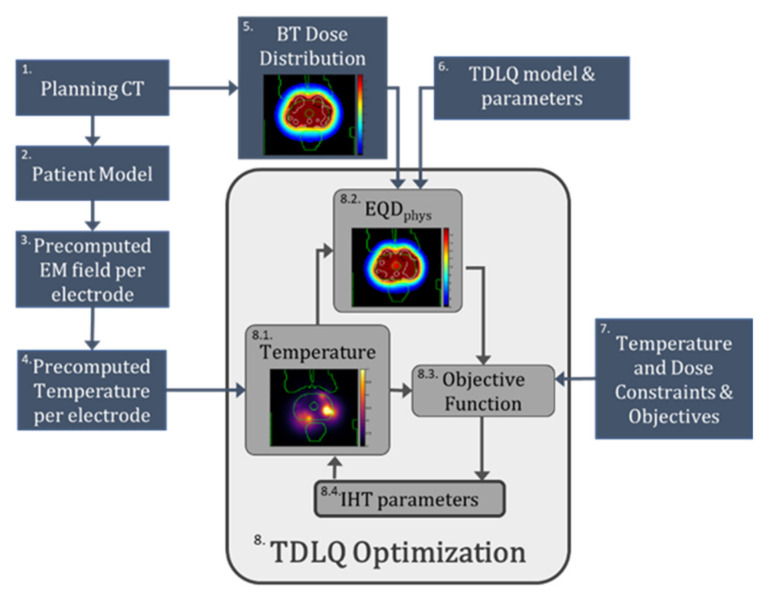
Graphical summary of the proposed optimization framework. 1. The planning CT is the initial input of the process. 2. The patient model is generated. 3. The EM field per electrode is precalculated. 4. The temperature distribution per electrode is precalculated. 5. The BT dose distribution is imported from the BT treatment planning software. 6. The TDLQ model is used for the calculation of the combined effect. 7. Both temperature and dose constraints and objectives are used for the optimization process. 8. The TDLQ optimization process optimizes the IHT parameters (8.4) that generate a temperature distribution (8.1) from which an EQD_phys_ distribution is generated (8.2). This EQD_phys_ distribution is used to compute the objective function, which needs to be minimized (8.3).
